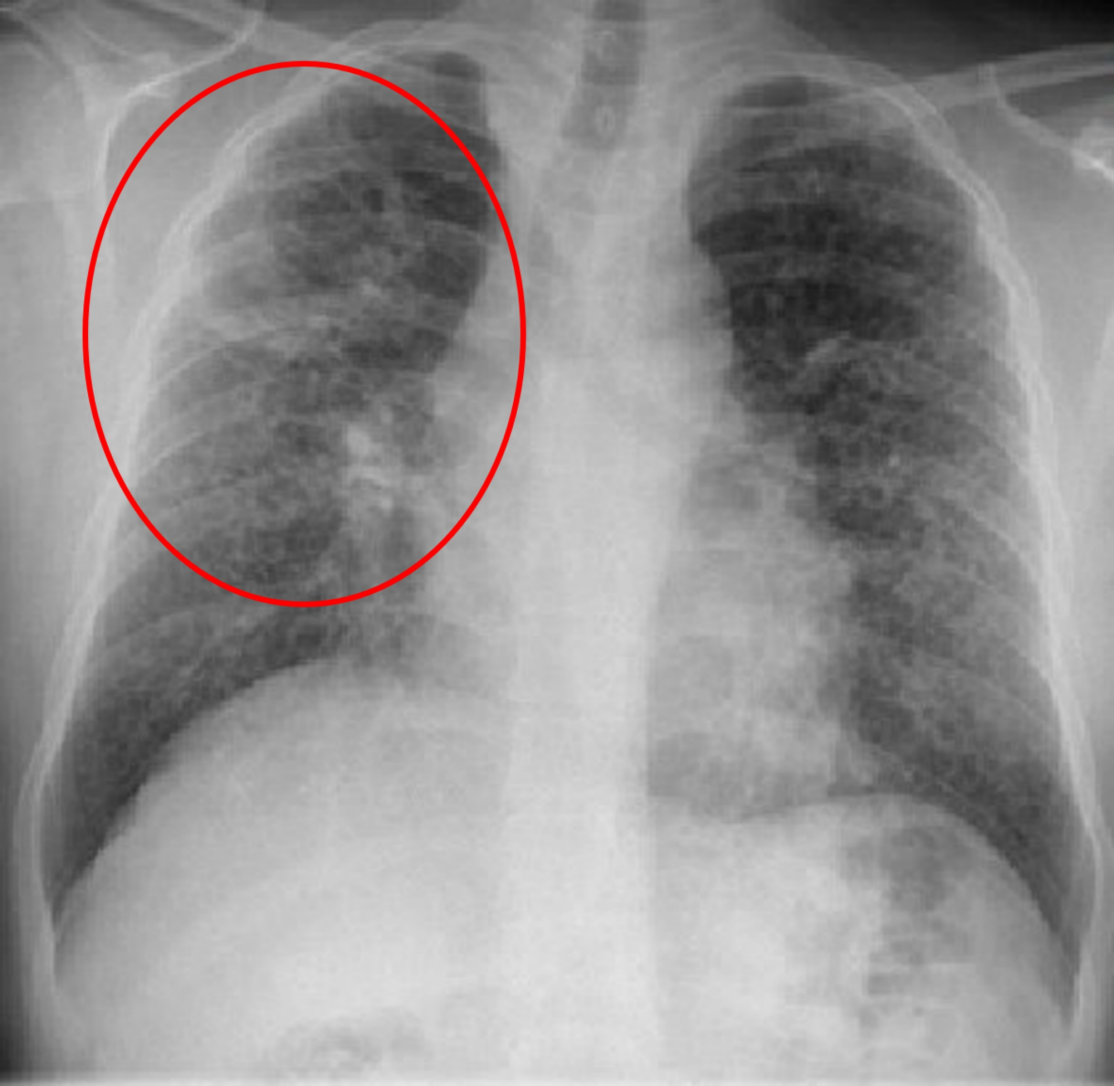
Chest radiograph showing right upper lobe cavitary lung lesion and diffuse nodular reticular interstitial opacities.
